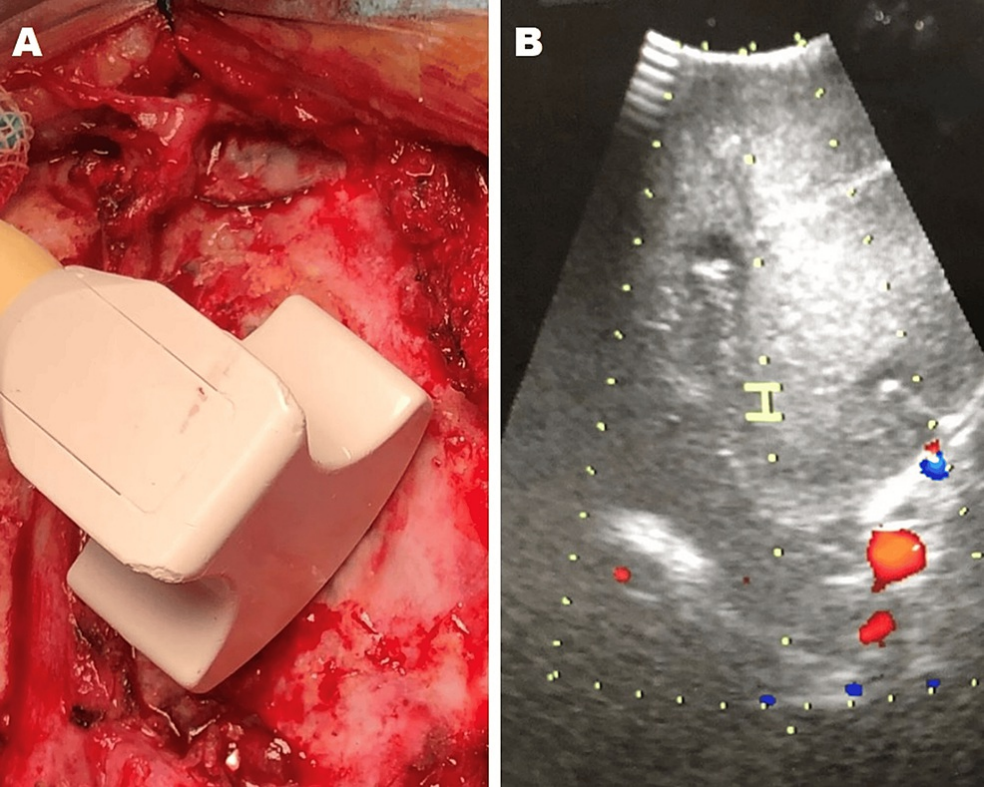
A 76-year-old patient with left frontal metastasis of clear cell renal carcinoma. (A) Placement of the transducer in the intraoperative, after having performed the craniotomy. (B) Intraoperative ultrasonography image after craniotomy where heterogeneous hyperechogenic intra-axial image is observed; tumor vessels are also observed
